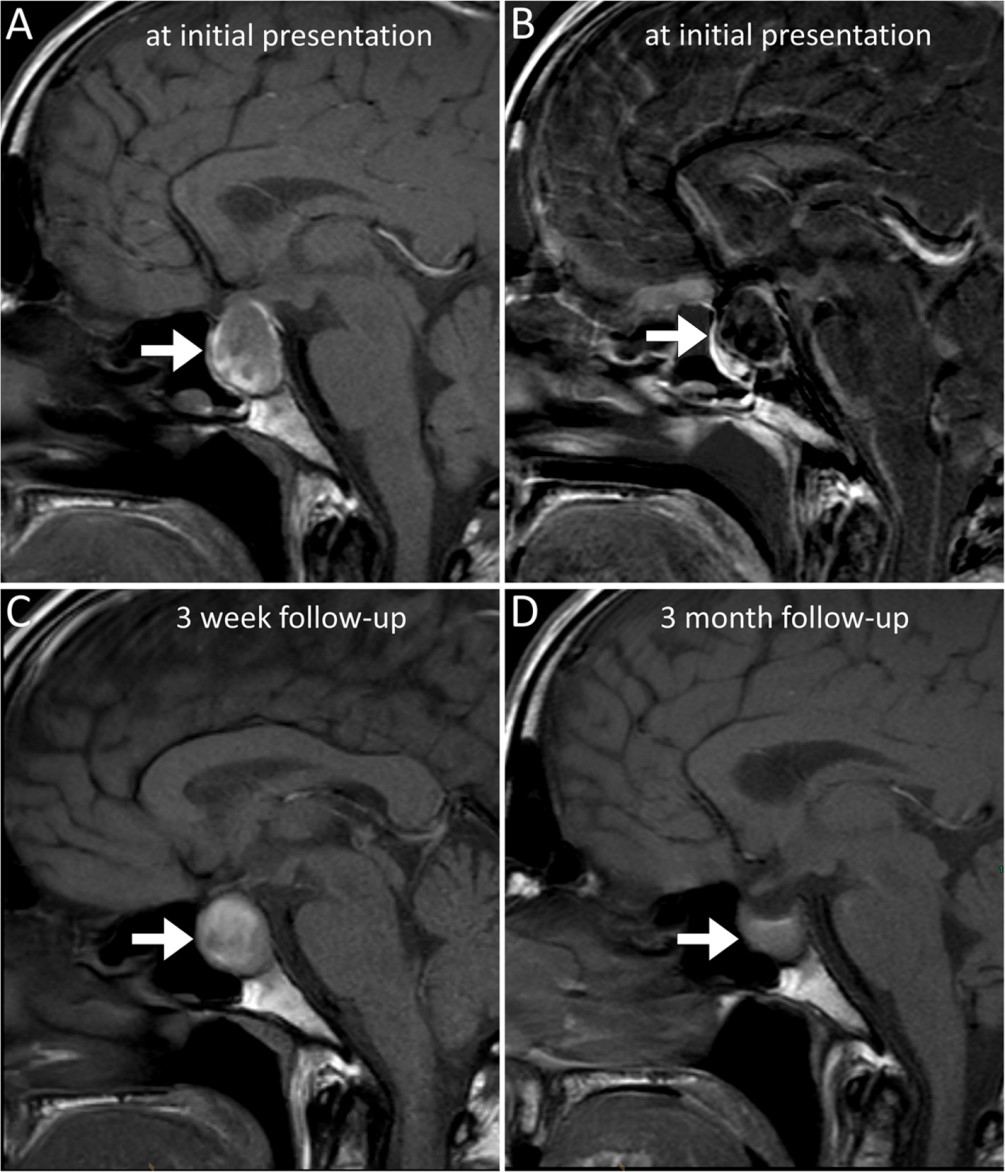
Magnetic resonance imaging of the sellar region. Magnetic resonance imaging (MRI) of the head disclosed a hemorrhagic intrasellar mass (arrow) with compression of the optic chiasm on initial presentation (a, native T1-weighted sequence in sagittal orientation shows a hyperintense signal in the periphery of the mass due to blood. b, subtraction of native and contrast-enhanced T1-weighted sequences shows only minimal peripheral and central enhancement). Follow-up MRI shows a shrinking and organized sellar hematoma after 3 weeks (c, native T1-weighted sequence) and 3 months, respectively (d, native T1-weighted sequence)
